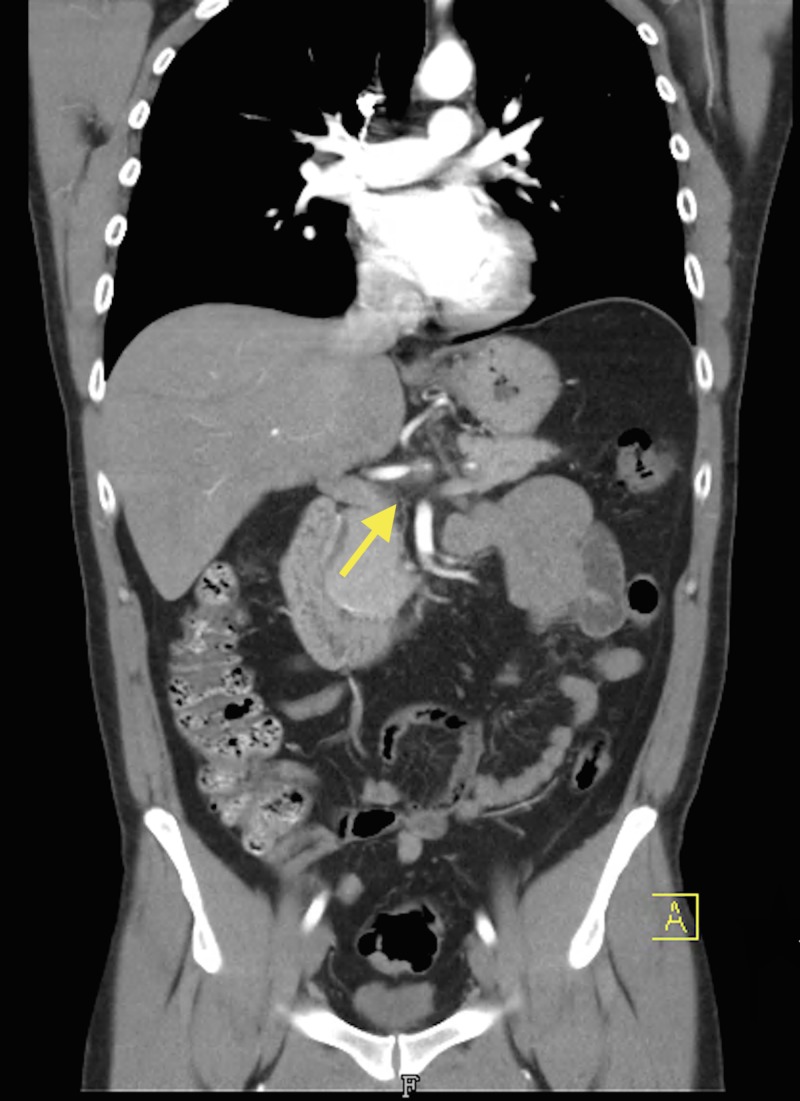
Coronal computed tomography angiography (CTA) of the abdomen and pelvis depicting celiac artery dissection with proximal hepatic artery stenosis (arrow)
